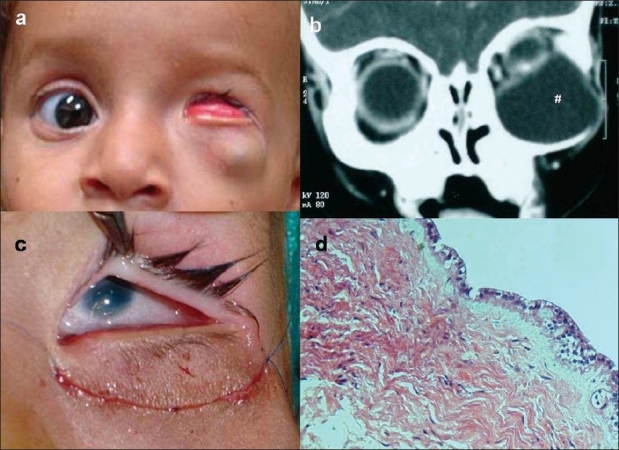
Cystic teratoma presenting with microphthalmos, (a) Clinical photograph, (b) Computed tomography showing a large cystic lesion occupying the inferior orbit and displacing the globe superiorly, (c) Intraoperative picture showing microphthalmos, (d) Cyst wall lined by cuboidal to columnar lining epithelium
